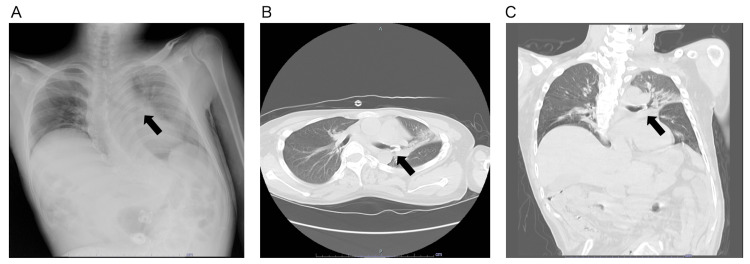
Chest radiograph and computed tomography (CT) images obtained during admission. A. A chest radiograph showing a foreign body (black arrow) in the left upper bronchus. B. Axial CT showing a U-shaped plastic foreign body in the left upper bronchus (black arrow). C. Coronal CT showing a U-shaped plastic foreign body in the left upper bronchus (black arrow).
